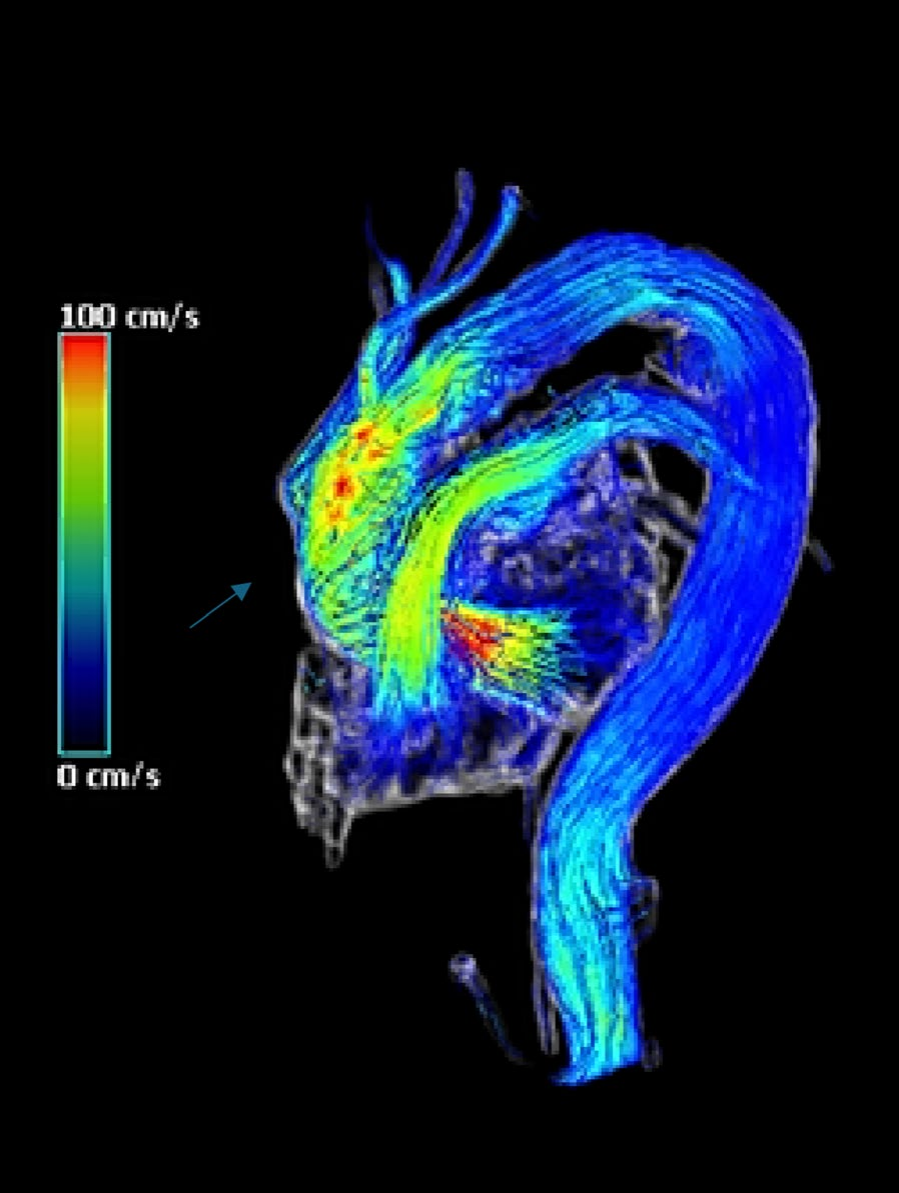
4D Flow MRI analysis: arrow: helical flow at the level of the ascending aorta, aortic arch and first portion of the aortic arch and progressive EL
